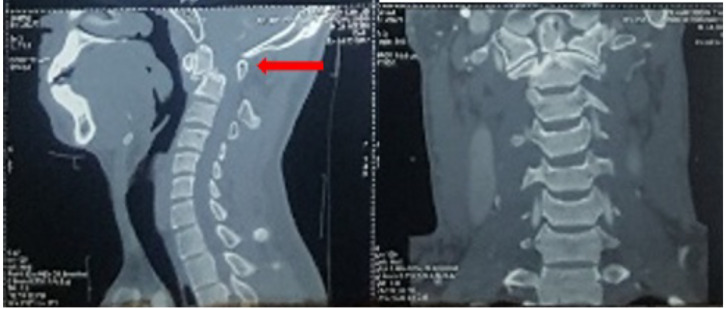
Sagittal-coronal planes CT scan of the cervical spine showed fracture at the axis (C2), specifically at the odontoid process, categorized as type II, based on Anderson and D'Alonzo classification (arrows).
